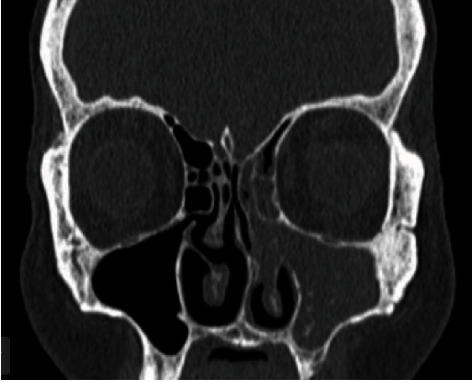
Frontal CT section showing the filling of the left maxillary sinus.
